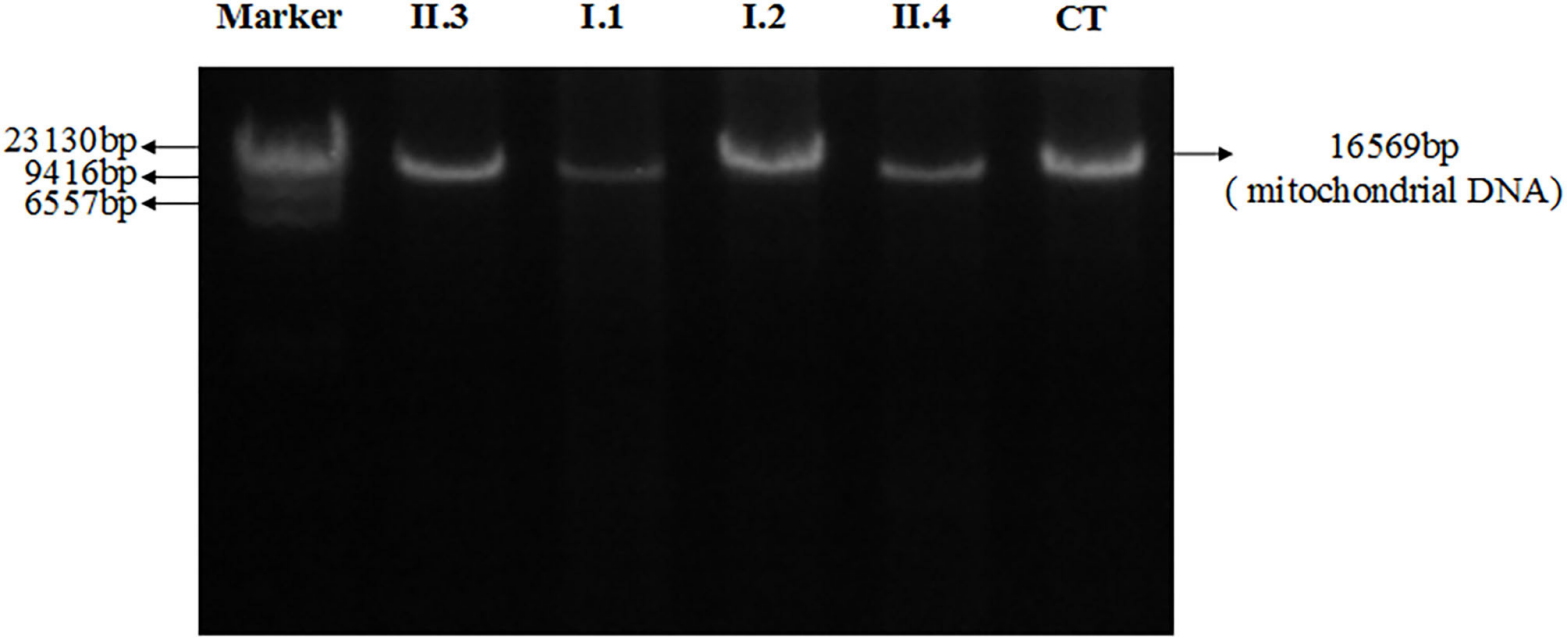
Electrophoresis results of amplified mitochondrial DNA by long-PCR of all patients and their parents. II.3: Proband; I.1: Father; I.2: Mother; II.4: Brother; CT: Control.
